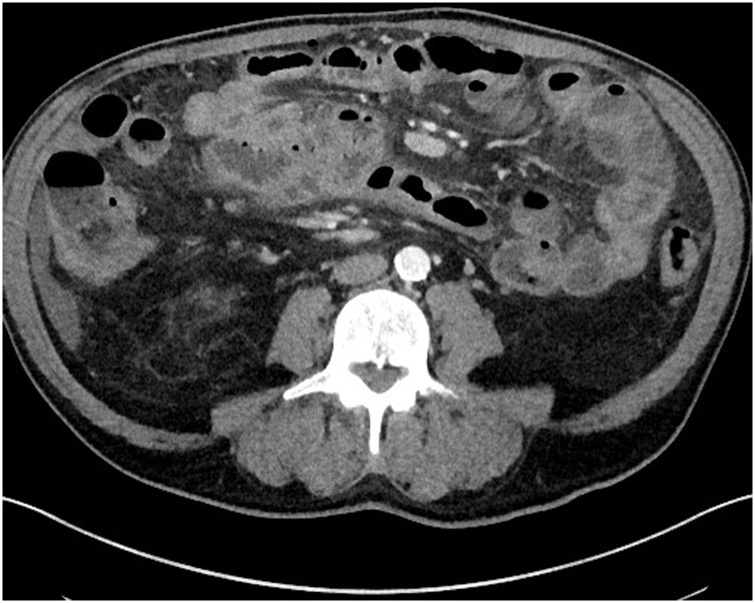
Axial CT scan showing adhesions between thick-walled segments of the small bowel and fat stranding.
